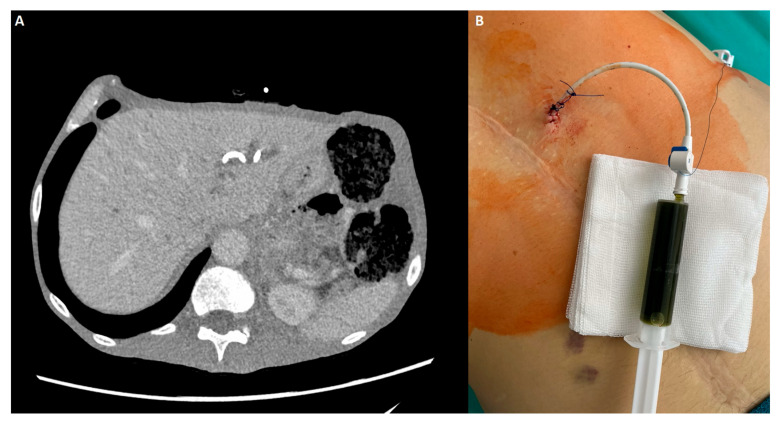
Percutaneous transhepatic biliary drain (PTBD) in the left hepatic duct (LHD) in patient with malignant biliary obstruction (MBO) after a failed endoscopic retrograde cholangiopancreatography (ERCP). (A) Computed tomography (CT) presenting a pigtail in the lumen of the biliary tract. (B) External drain with bile in the syringe collected straight after the procedure.
